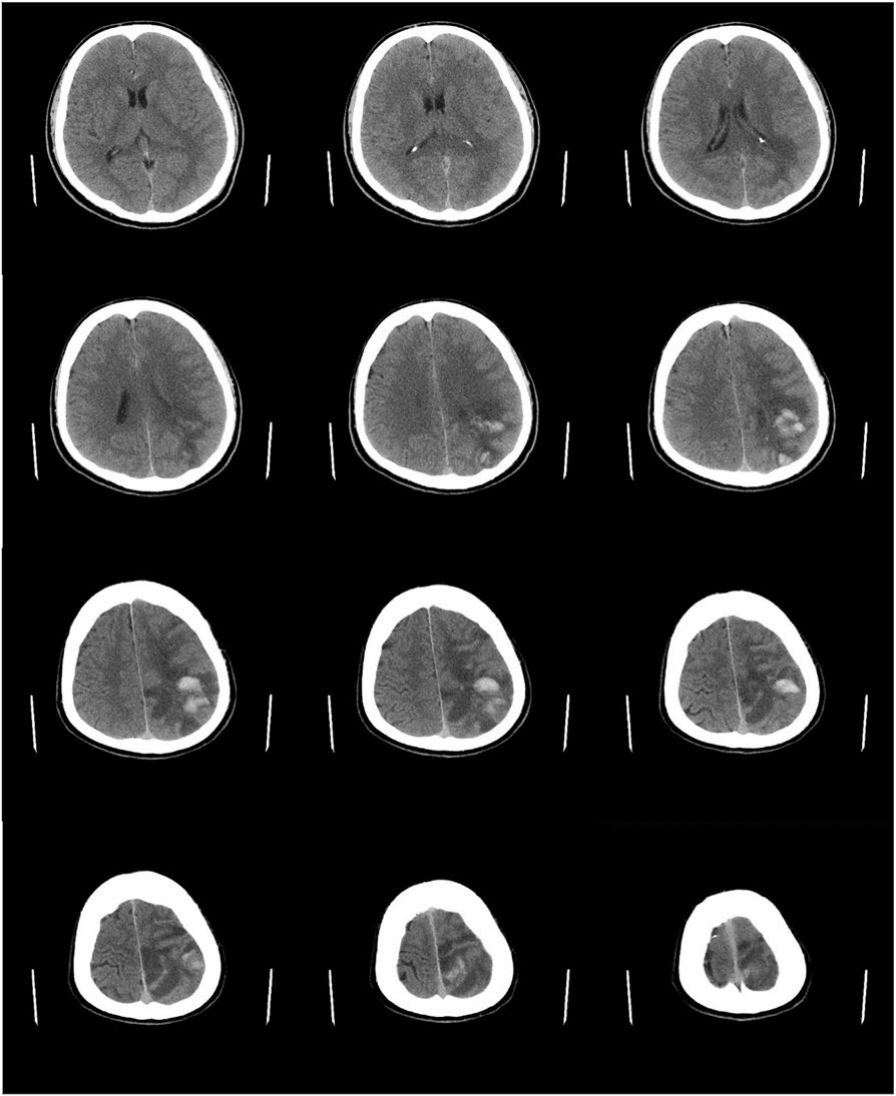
Computed tomography scan of the brain axial cuts shows a hypodense mixed with hyperintense lesion consist with cerebral infarction and hemorrhage transformation in the left subcortical and corona radiata region. The hemorrhage was more than 30% of the infarct area
